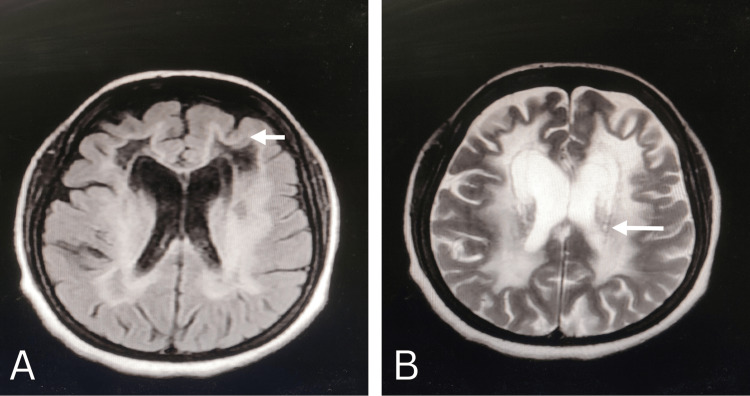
Magnetic resonance imaging findings in classic vanishing white matter (VWM) disease. (A): Axial T1-weighted image showing the diffuse abnormality (hypointense) of the white matter without involving grey matter (B): Axial T2-weighted image shows the hyperintense white matter projecting similar signals like cerebrospinal fluid.
